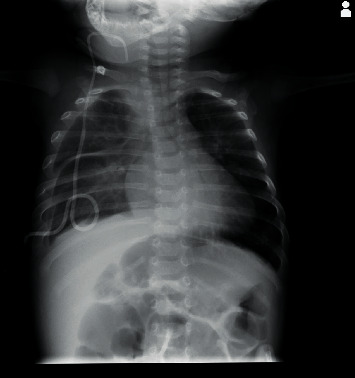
Chest radiograph at discharge from the neonatal care unit.
